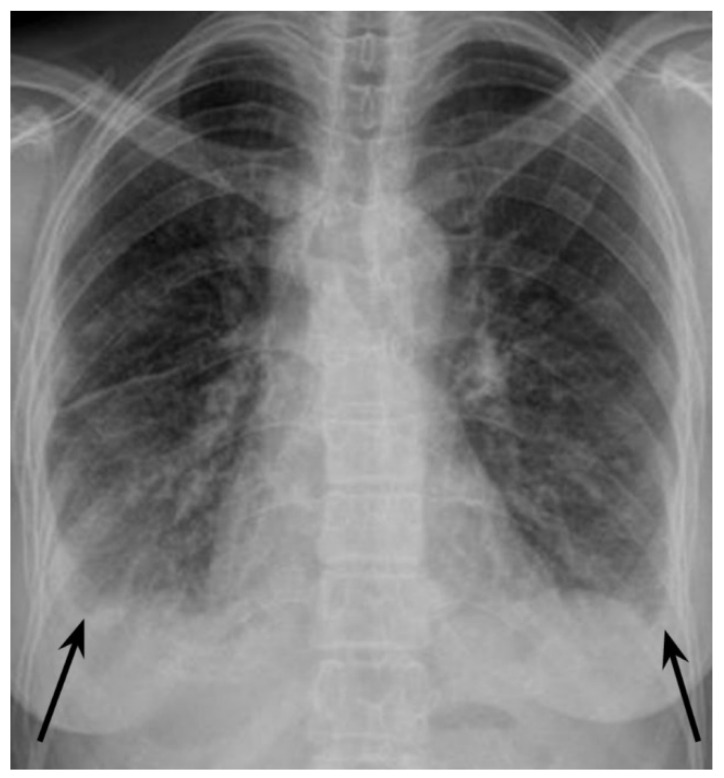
Infection with human immunodeficiency virus (HIV) is the strongest known risk factor for active tuberculosis (TB), and the risk of developing active TB in people living with HIV (PLWH) is 15–22 times higher than in people without HIV [1]. Active TB may develop at any stage of HIV infection, but the risk correlates negatively with CD4^+^ cells count. TB is the leading cause of morbidity, hospitalisations, and mortality in PLWH [1]. There were 214,000 deaths due to TB among HIV-positive people in 2020 worldwide, which accounted for 31.5% of all HIV-related deaths [1,2]. Therefore, it is recommended to screen for TB in HIV-positive patients, and for HIV infection in newly diagnosed TB patients [3,4,5]. Around 16% of all PLWH do not know that they are infected with HIV [1], and about 25% of incident HIV patients present to care with advanced disease [3]. Immunosuppression caused by HIV infection affects clinical and radiologic presentation of TB. Atypical TB presentation is often observed in the late stages of HIV infection [6,7,8,9]. Such atypical TB presentation in a person with HIV infection not yet diagnosed, may be challenging, as described below. A 42-year-old woman of Indian origin was referred to a respiratory medicine department after her chest X-ray (Figure 1) revealed nodular opacifications in the lungs and bilateral pleural effusion (arrows). The patient had a 4-month history of unspecific chest and feet pains, mild dry cough, fatigue, reduced appetite, and body weight loss of 6 kg. She denied dyspnoea, sputum expectoration, haemoptysis, night sweats, or fever. On admission to the hospital, she was in good condition, her vital signs were normal, BMI was 19.2. There was no palpable peripheral lymphadenopathy or oedema; the vesicular breathing sound was reduced bibasiliary on chest auscultation. Blood tests showed elevated CRP—109.4 (N:<5) mg/L and ERS—120 (N: < 12), normal procalcitonin, normal leukocyte and neutrophil counts, decreased lymphocyte count—0.84 × 10^3^ (N:1.18 × 10^3^–3.74 × 10^3^) cells/mm^3^.
